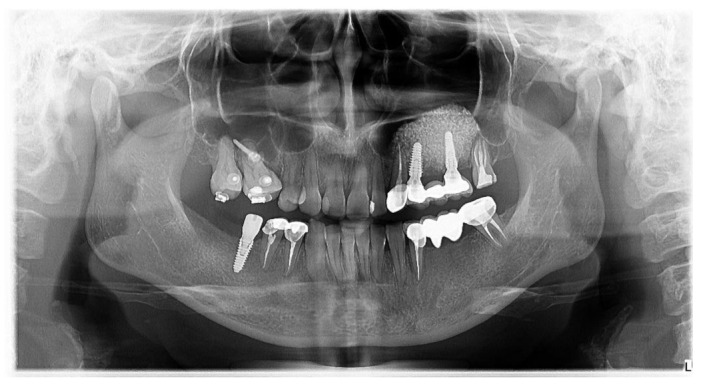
Postoperative panoramic x-ray at 1-year after loading.
